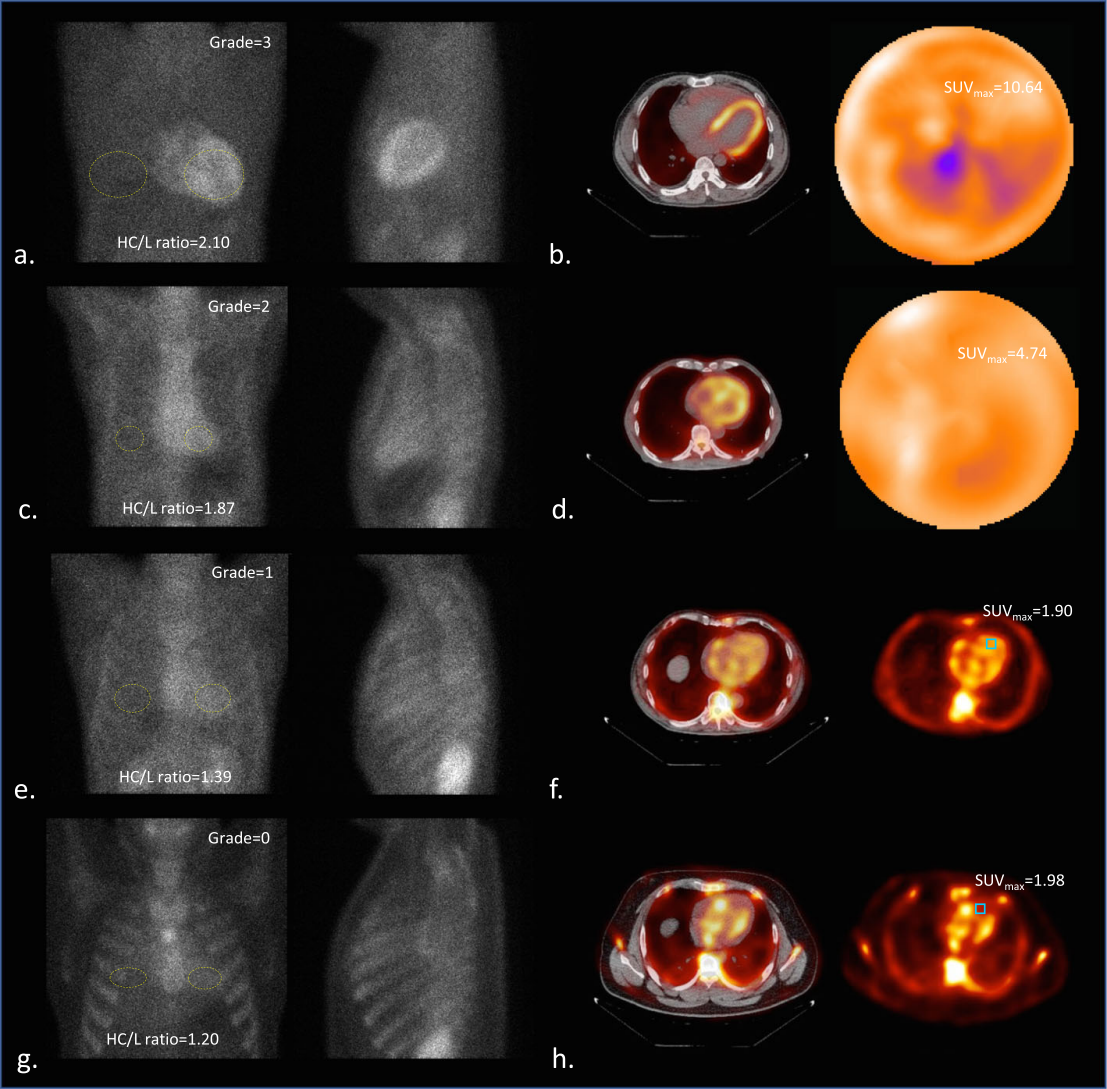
Representative images of ^99m^Tc-PYP planar and quantitative SPECT. a Grade = 3 in Perugini visual scores with H/CL ratio = 2.10 and SUV_max_ = 10.64 g/ml in b. c Grade = 2 with H/CL ratio = 1.87 and SUV_max_ = 4.74 g/ml in d. e Grade = 1 with H/CL ratio = 1.39 and SUV_max_ = 1.90 g/ml in f. e Grade = 0 with H/CL ratio = 1.20 and SUV_max_ = 1.98 g/ml in h
